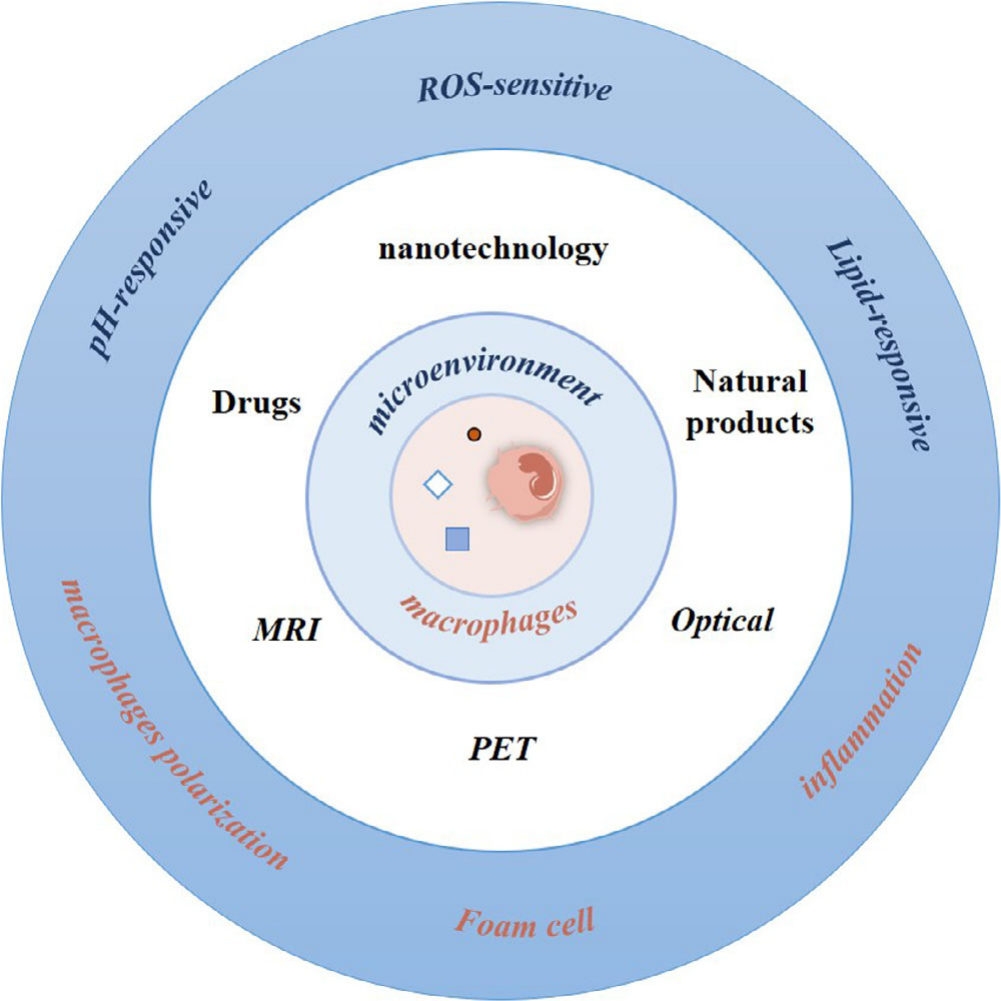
Diagnostic and therapeutic maps of the atherosclerotic plaque microenvironment and macrophages. It sequentially shows three therapeutic modalities (drugs, natural products and nanotechnology) and three imaging modalities (magnetic resonance imaging [MRI], positron emission tomography [PET] and optical) for microenvironment and macrophages. The outermost circle shows the different treatment strategies.
